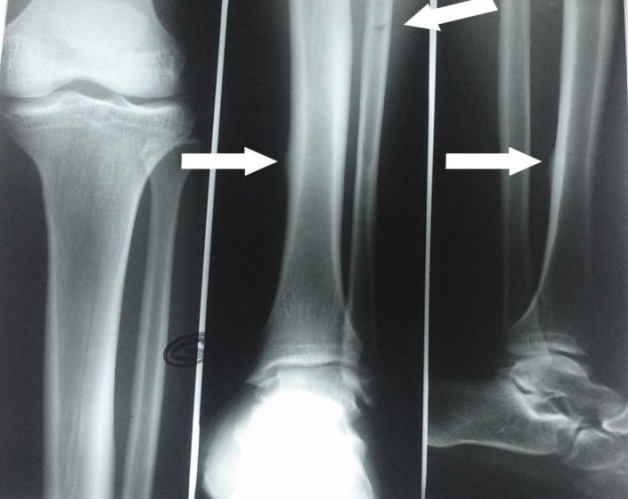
Caporal de 24 ans présentant une douleur du tiers inférieur de la jambe gauche au cours d'une marche pendant un stage militaire: radiographie de la jambe gauche de face et de profil montrant une fracture corticale pure médiale du tiers inférieur de la diaphyse tibiale
